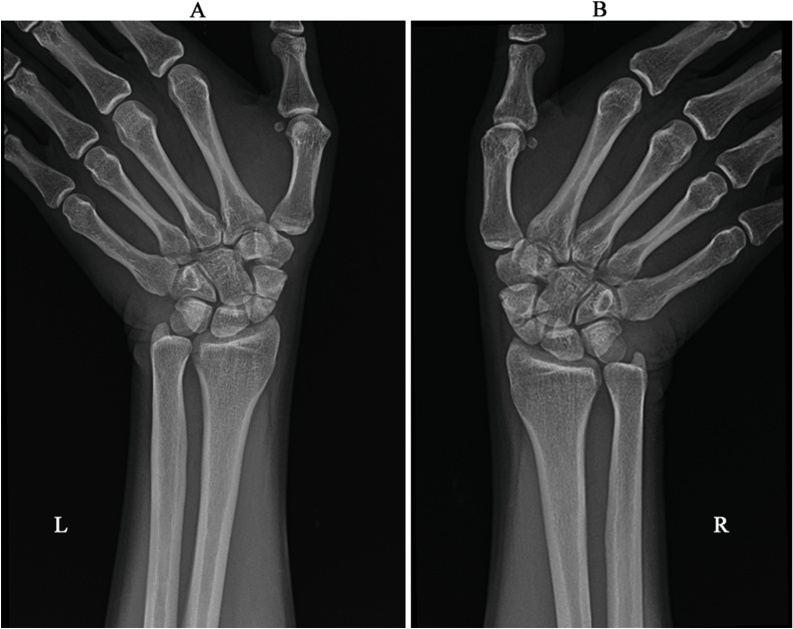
X-rays showing anteroposterior views with ulnar deviation of both writs showing bilateral scaphoid fractures.
